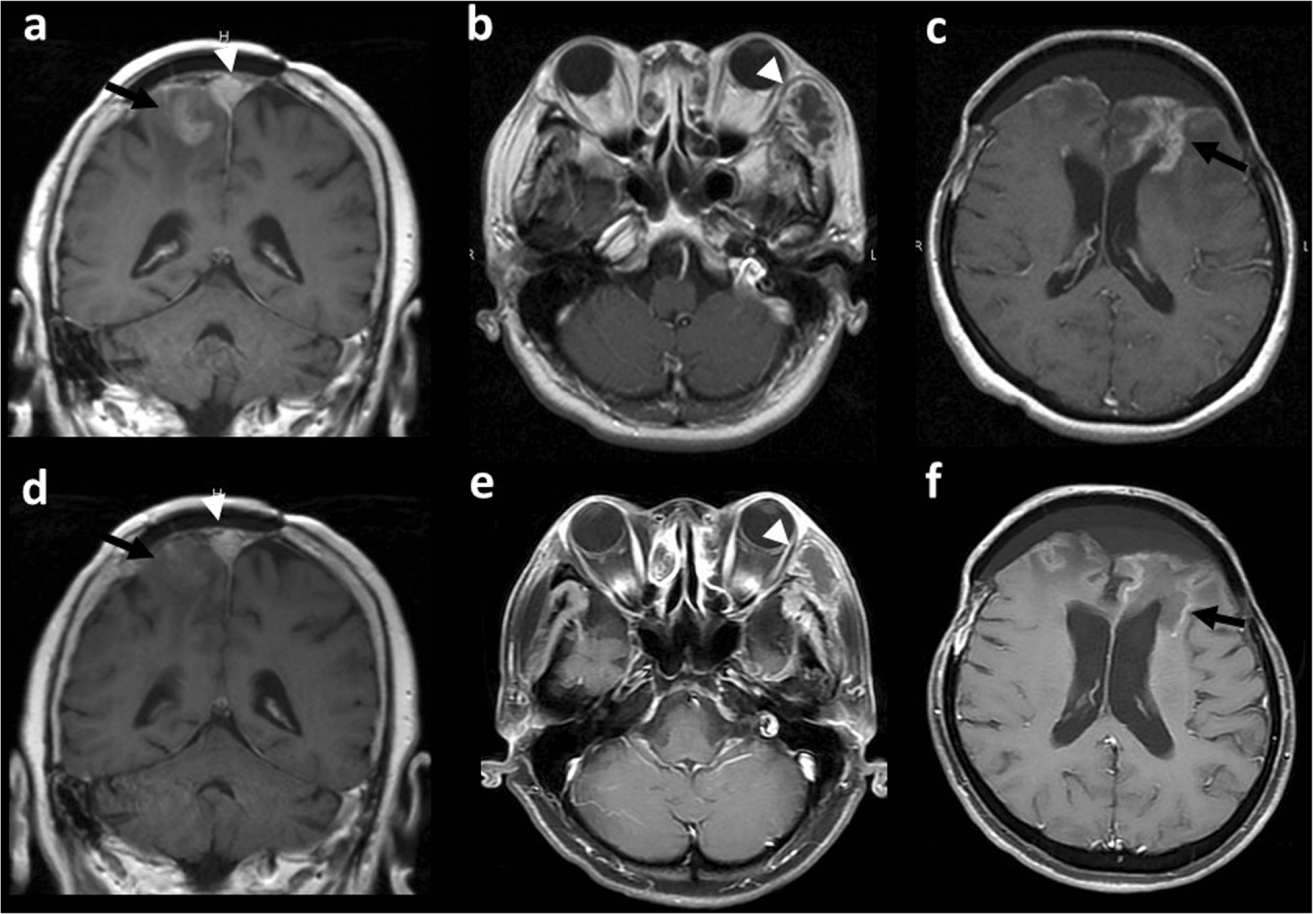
MR images before and after bevacizumab in Case 3 (before, a; after, d) and Case 6 (before, b, c; after, e, f). Intraparenchymal radiation necrosis decreased to −65 % and −51 % after bevacizumab in Cases 3 and 6, respectively (black arrows). Regarding post-irradiated tumors, Tumor 9 (Case 3) (a, d, arrowhead) did not change (0 %) and Tumor 13 (Case 6) (b, e, arrowhead) showed 65 % decrease after bevacizumab
